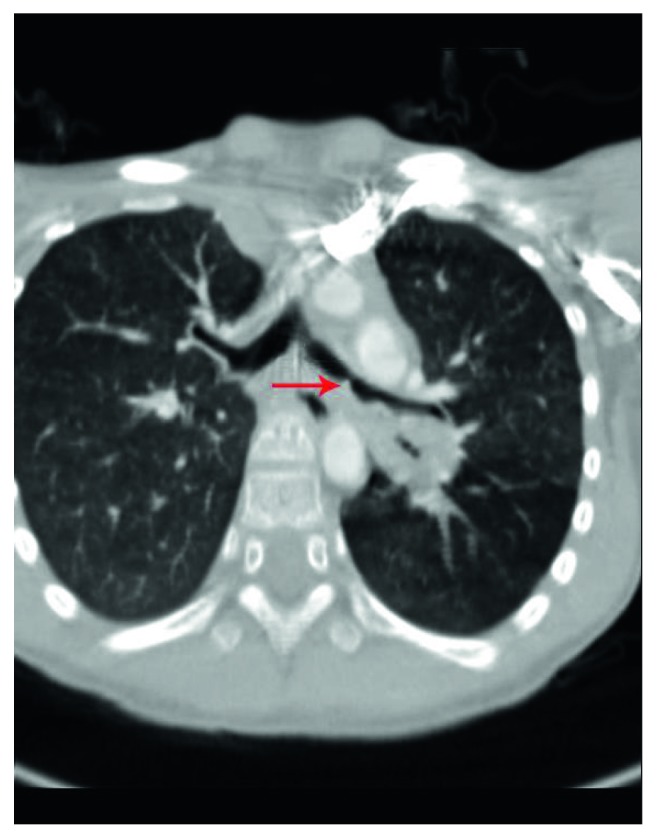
Computed tomography revealed a soft tissue density (red arrow) at the left lower lobe bronchus with narrowing of the left main bronchus and emphysema of the left lower lobe.
